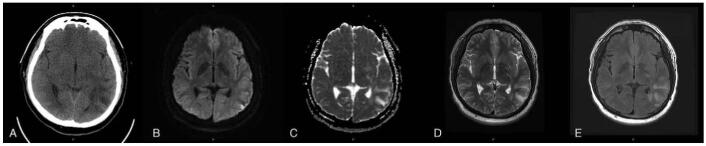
Cerebral computed tomography (A) disclosed a hypodense lesion in the left parieto-temporal lobe. Initially, it was difficult to differentiate between acute ischemic stroke and vasogenic edema. Cerebral MRI disclosed a slight hyperintense signal on diffusion-weighted imaging (B) in the left parieto-temporal lobe that was not accompanied by a decreased signal on apparent diffusion coefficient (C). Also, we identified hyperintensity of T2-weighted imaging (D) and fluid-attenuated inversion recovery (E) in the same area, which suggested the presence of vasogenic cerebral edema rather than cerebral infarction. MRI = magnetic resonance imaging.
